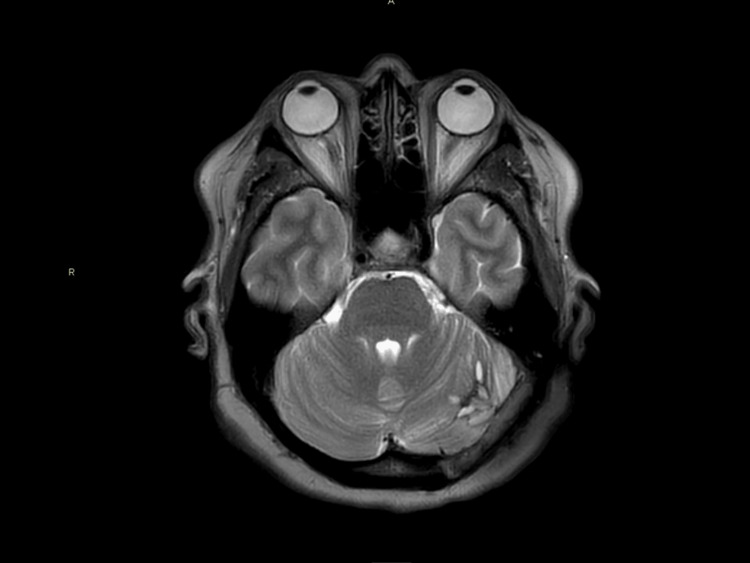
Postoperative MRI: T2 sequence showing no residual tumor and disappearance of the mass effect. MRI: Magnetic resonance imaging
